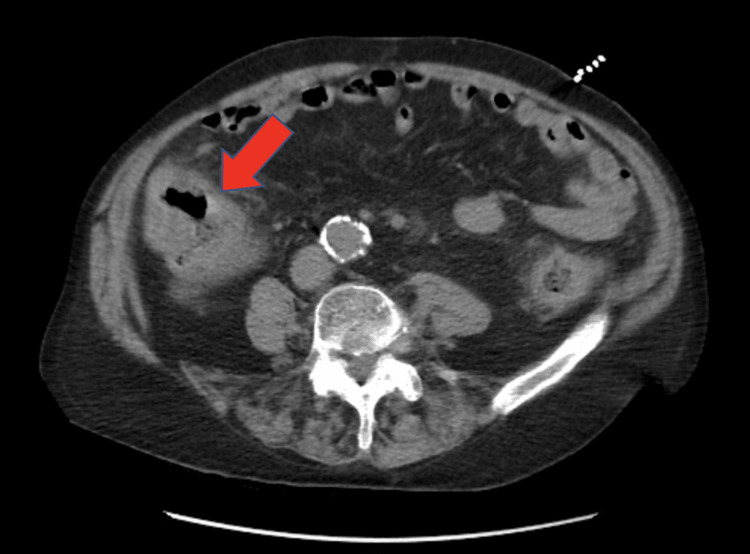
Computed tomography (CT) of the abdomen/pelvis without contrast on admission showing generalized large bowel inflammation with wall thickening and infiltration of the adjacent fatty tissue consistent with generalized colitis or inflammatory bowel disease
